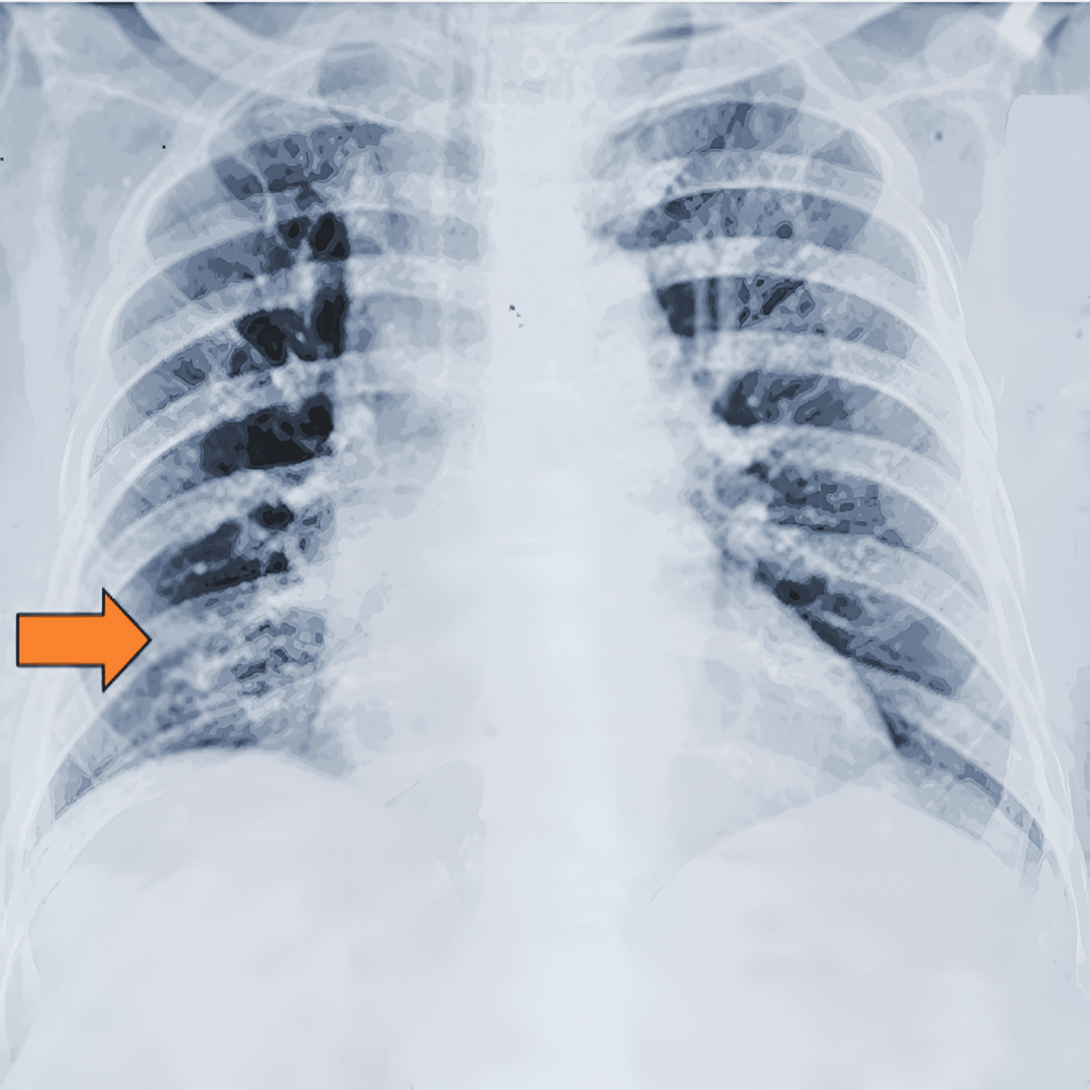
Chest X-ray showing right lower zone consolidation (orange arrow).
